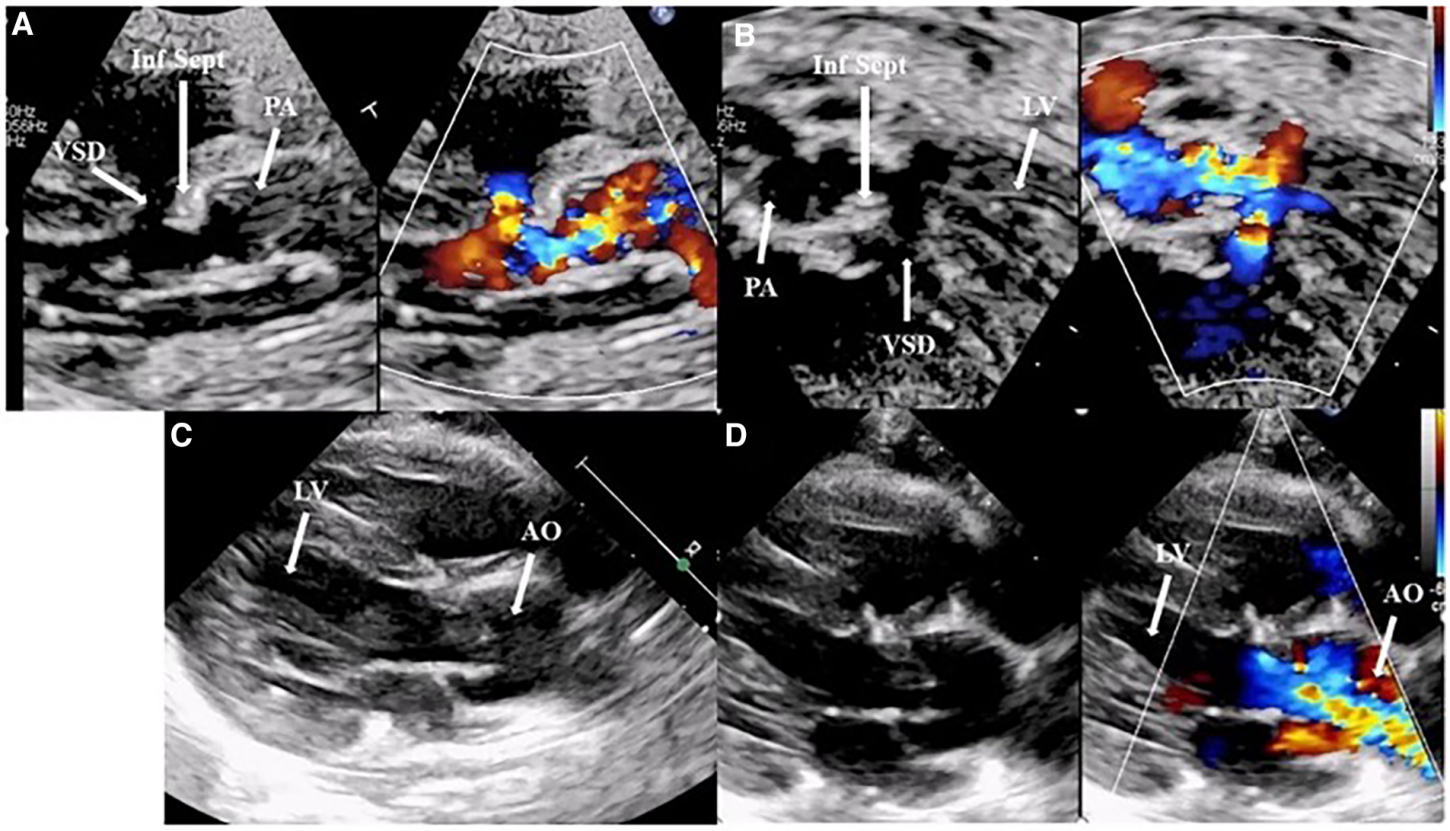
(A,B) A preoperative transthoracic echocardiogram: a parasternal long-axis view and five-chamber view with and without a color Doppler, highlighting the posterior deviation of the infundibular septum causing subvalvular and valvar pulmonary obstruction and the restrictive interventricular septal defect. Images (C,D), A postoperative transthoracic echocardiogram: a parasternal long-axis view without and with a color Doppler, showing an excellent surgical outcome after an arterial switch operation, closure of the interventricular defect, and resection of the infundibular septum. AO, aorta; Inf Sept, infundibular septum; LV, left ventricle; PA, pulmonary artery.
